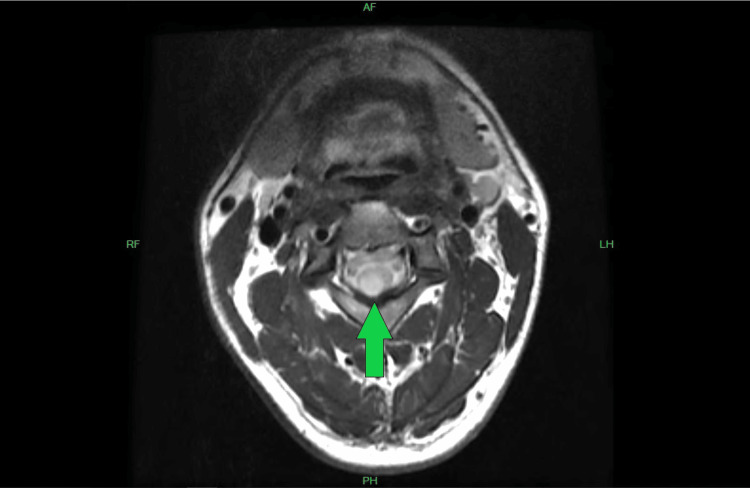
Cervical Spine 3 Tesla MRI Axial View Without Intravenous Contrast There was an abnormal signal noted within the cervical spinal cord from the C2-C3 level to the top of the C4 level spanning 24 mm in height, 8.5 mm in transverse dimension, and 7.7 mm in anterior/posterior dimension. This had the appearance of expanding past the posterior right aspect of the spinal cord (green arrow).
